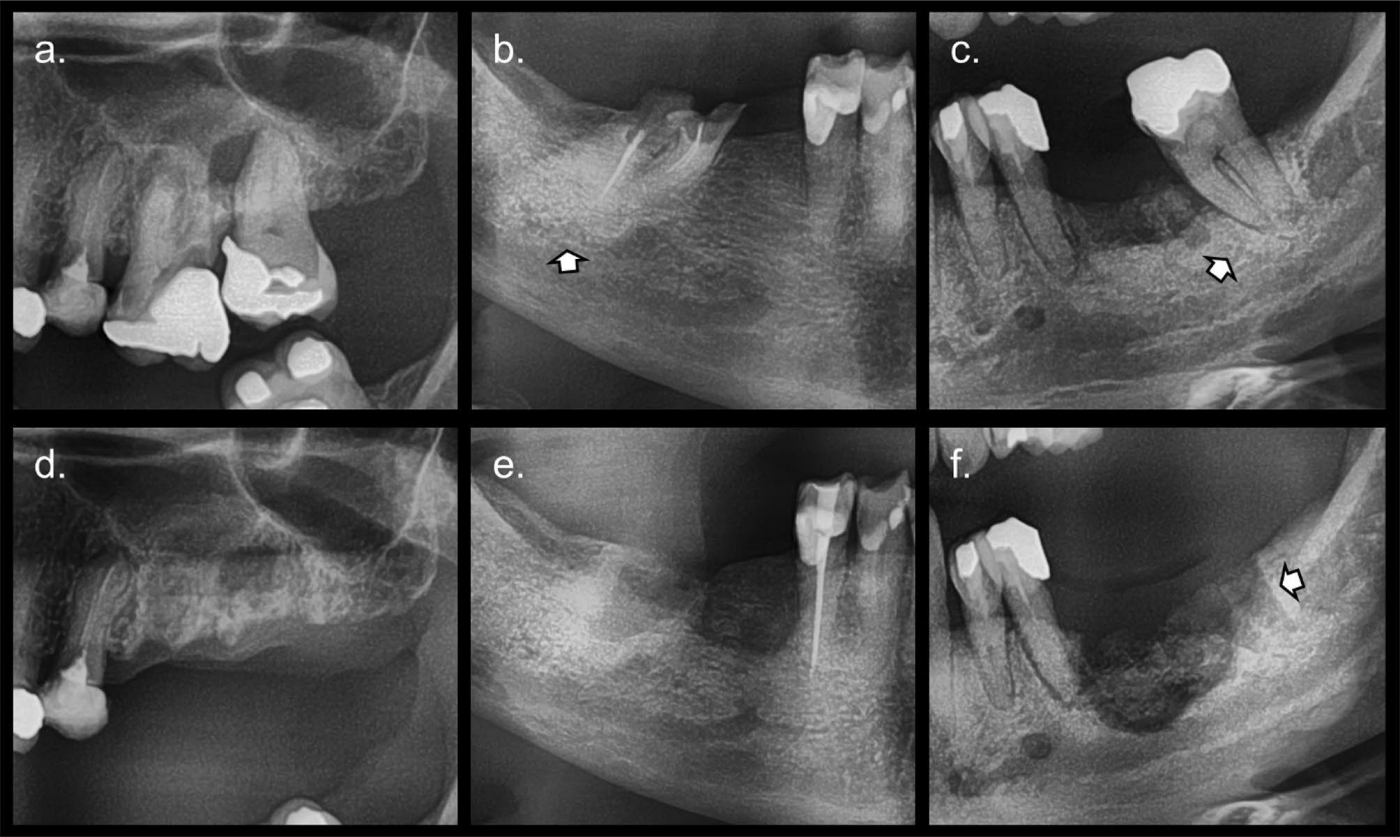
Cropped panoramic images showing pre-operative sites (a–c) and their respective post-operative evolution (d–f). Pre-operative images show presence of risk factors, teeth without endodontic treatment and with periapical lesion (a, c), incomplete endodontic filling in length and presence of caries (b), and sclerotic bone pattern (b, c; white arrow). All extraction sites developed osteonecrosis, showing a heterogenous bone pattern (d, f), a sclerotic bone pattern (e), visible extraction socket (e, f), persistence of the lamina dura (f; white arrow), and sequester formation (f).
